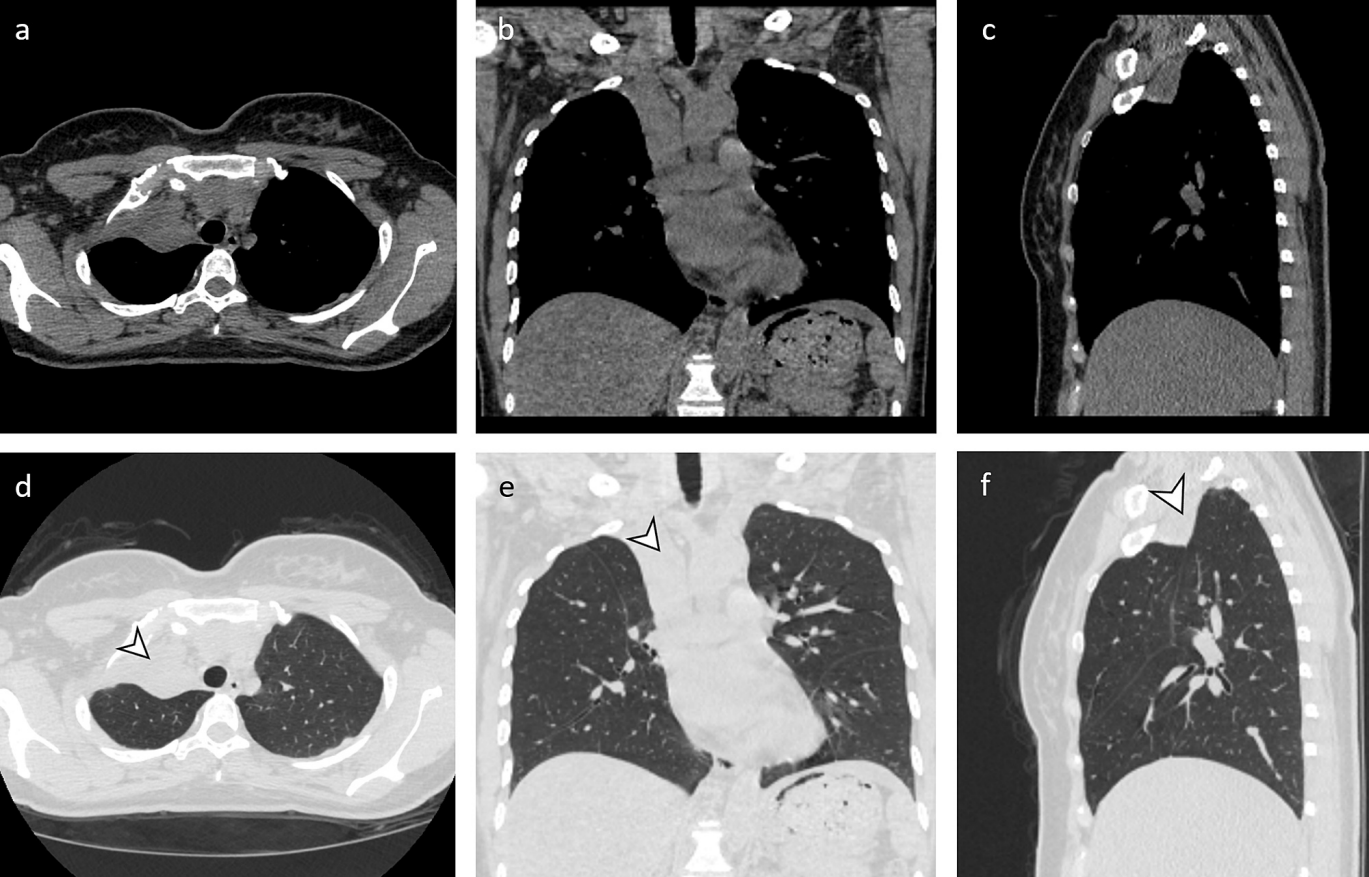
Post-treatment CT chest. Axial (a), coronal (b), and sagittal (c) non-contrast CT chest images in soft tissue window as well as axial (d), coronal (e), and sagittal (f) images in lung window demonstrating complete right upper lobe atelectasis (arrowhead). No right upper lobe bronchial or airspace aeration is present. The remaining portions of the lungs are clear.
